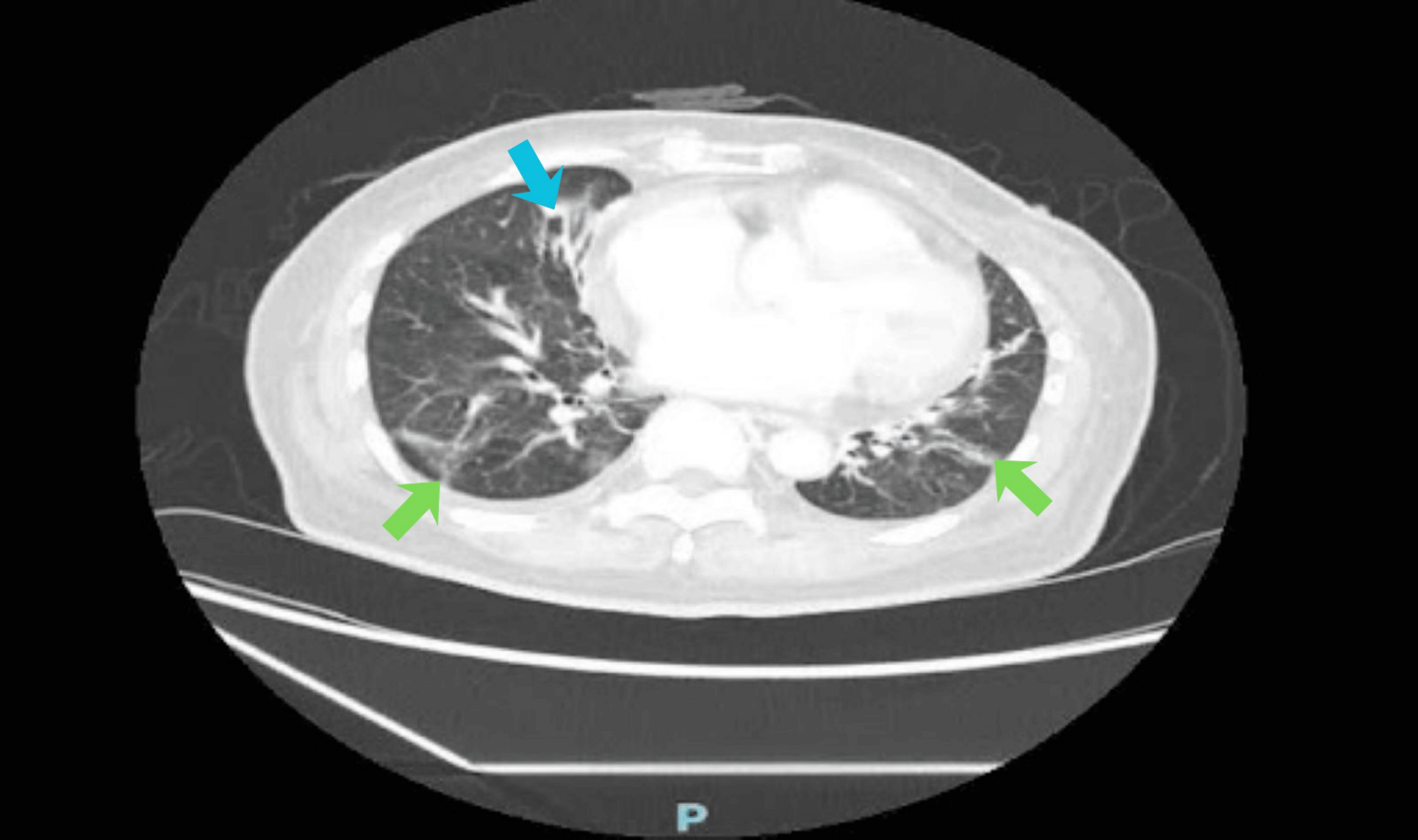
Standard computed tomography (CT) thorax Cross-sectional imaging of lungs; evidence of bronchiectatic lung changes (blue arrow) in the right middle lobe with bilateral patchy ground glass changes (green arrows).
